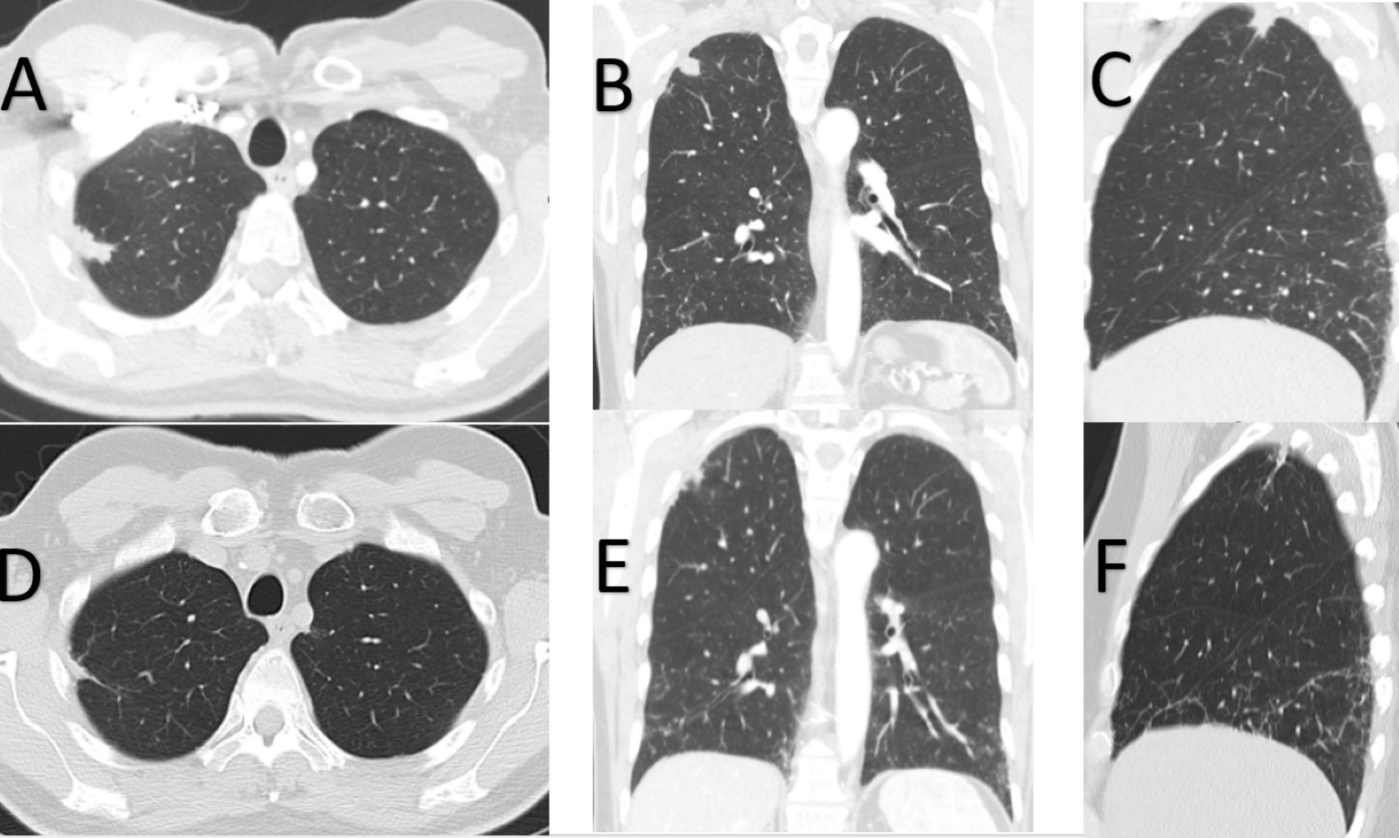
Initial six-month follow-up chest CT (A) axial, (B) coronal, and (C) sagittal views showing a new mass measuring 3.2 cm x 2.1 cm at the lateral aspect of the right pulmonary apex, contiguous with adjacent thickened pleura with reticulonodular and fibrotic changes in the bilateral lower lobes; Six-month follow-up post-lung biopsy chest CT (D) axial, (E) coronal, (F) sagittal views revealing regression of the lesion, with only chronic scarring observed from the prior lung biopsy, and stable reticulonodular and fibrotic changes in the bilateral lower lobes.
